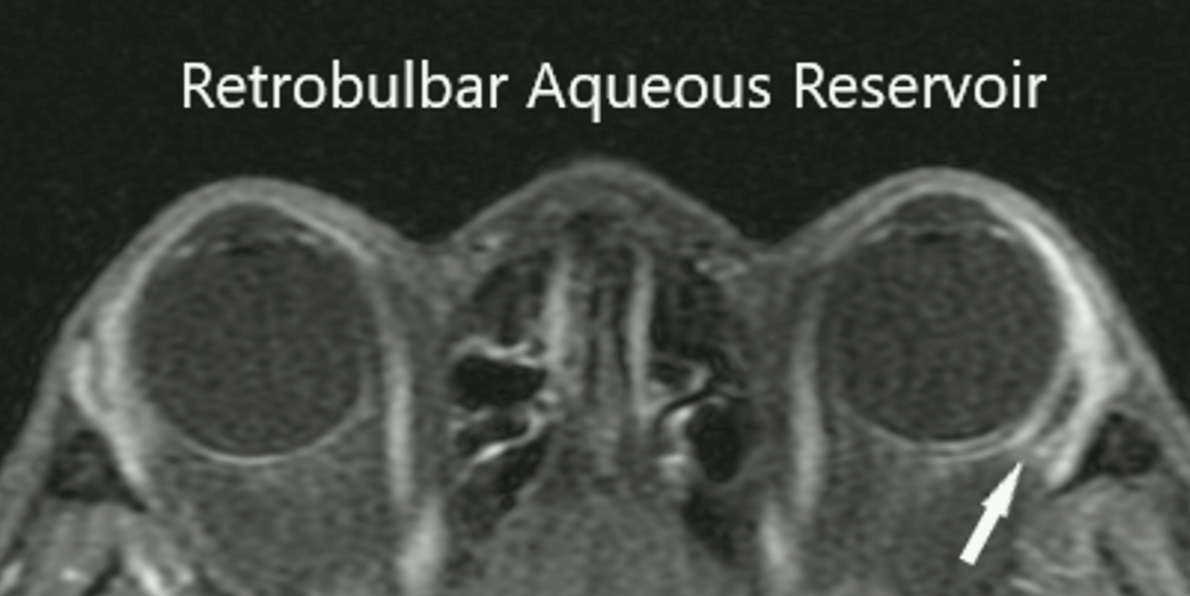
MRI showing aqueous in retrobulbar space.
